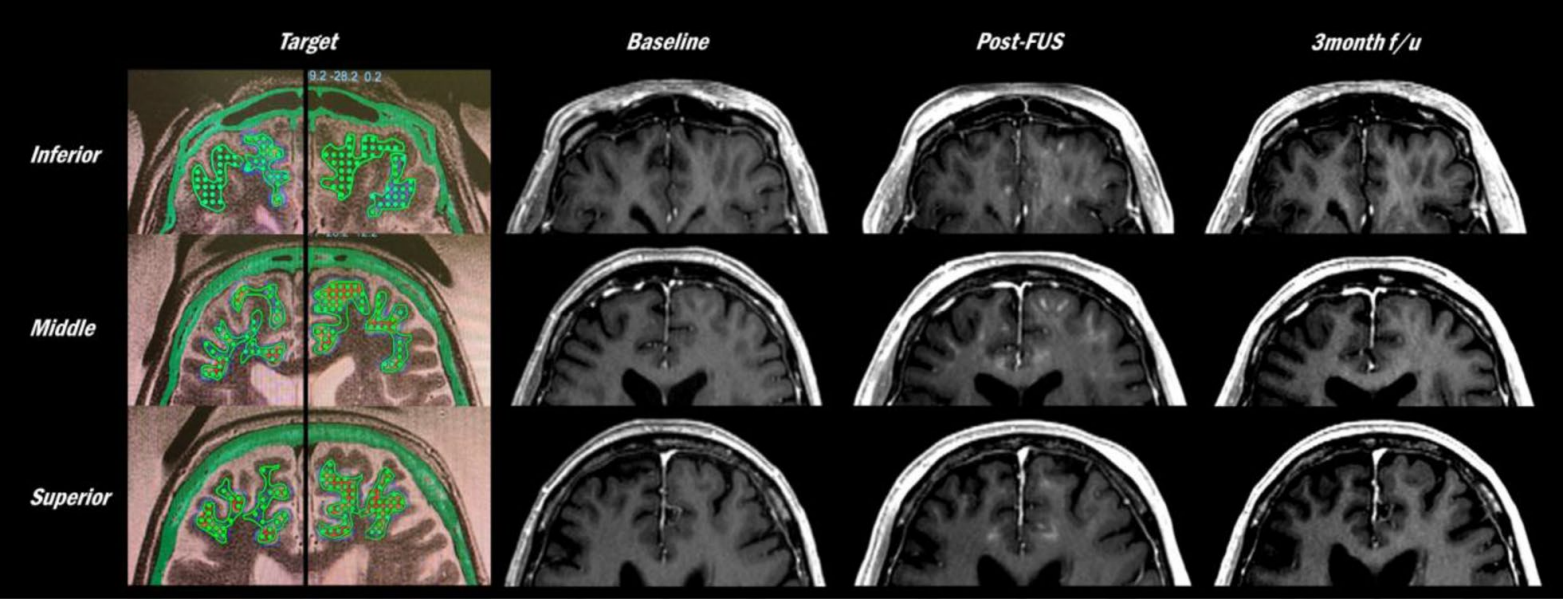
The targets and MRI demonstration of BBB opening and closure in Patient 3. Immediately post-FUS gadolinium-enhanced T1 MRI showed that parenchyme at target was well enhanced, indicating the successful BBB opening. Afterwards, this area did not show enhancement in MRI at 3 months post-procedure, indicating that the BBB was  temporarily opened and closed
